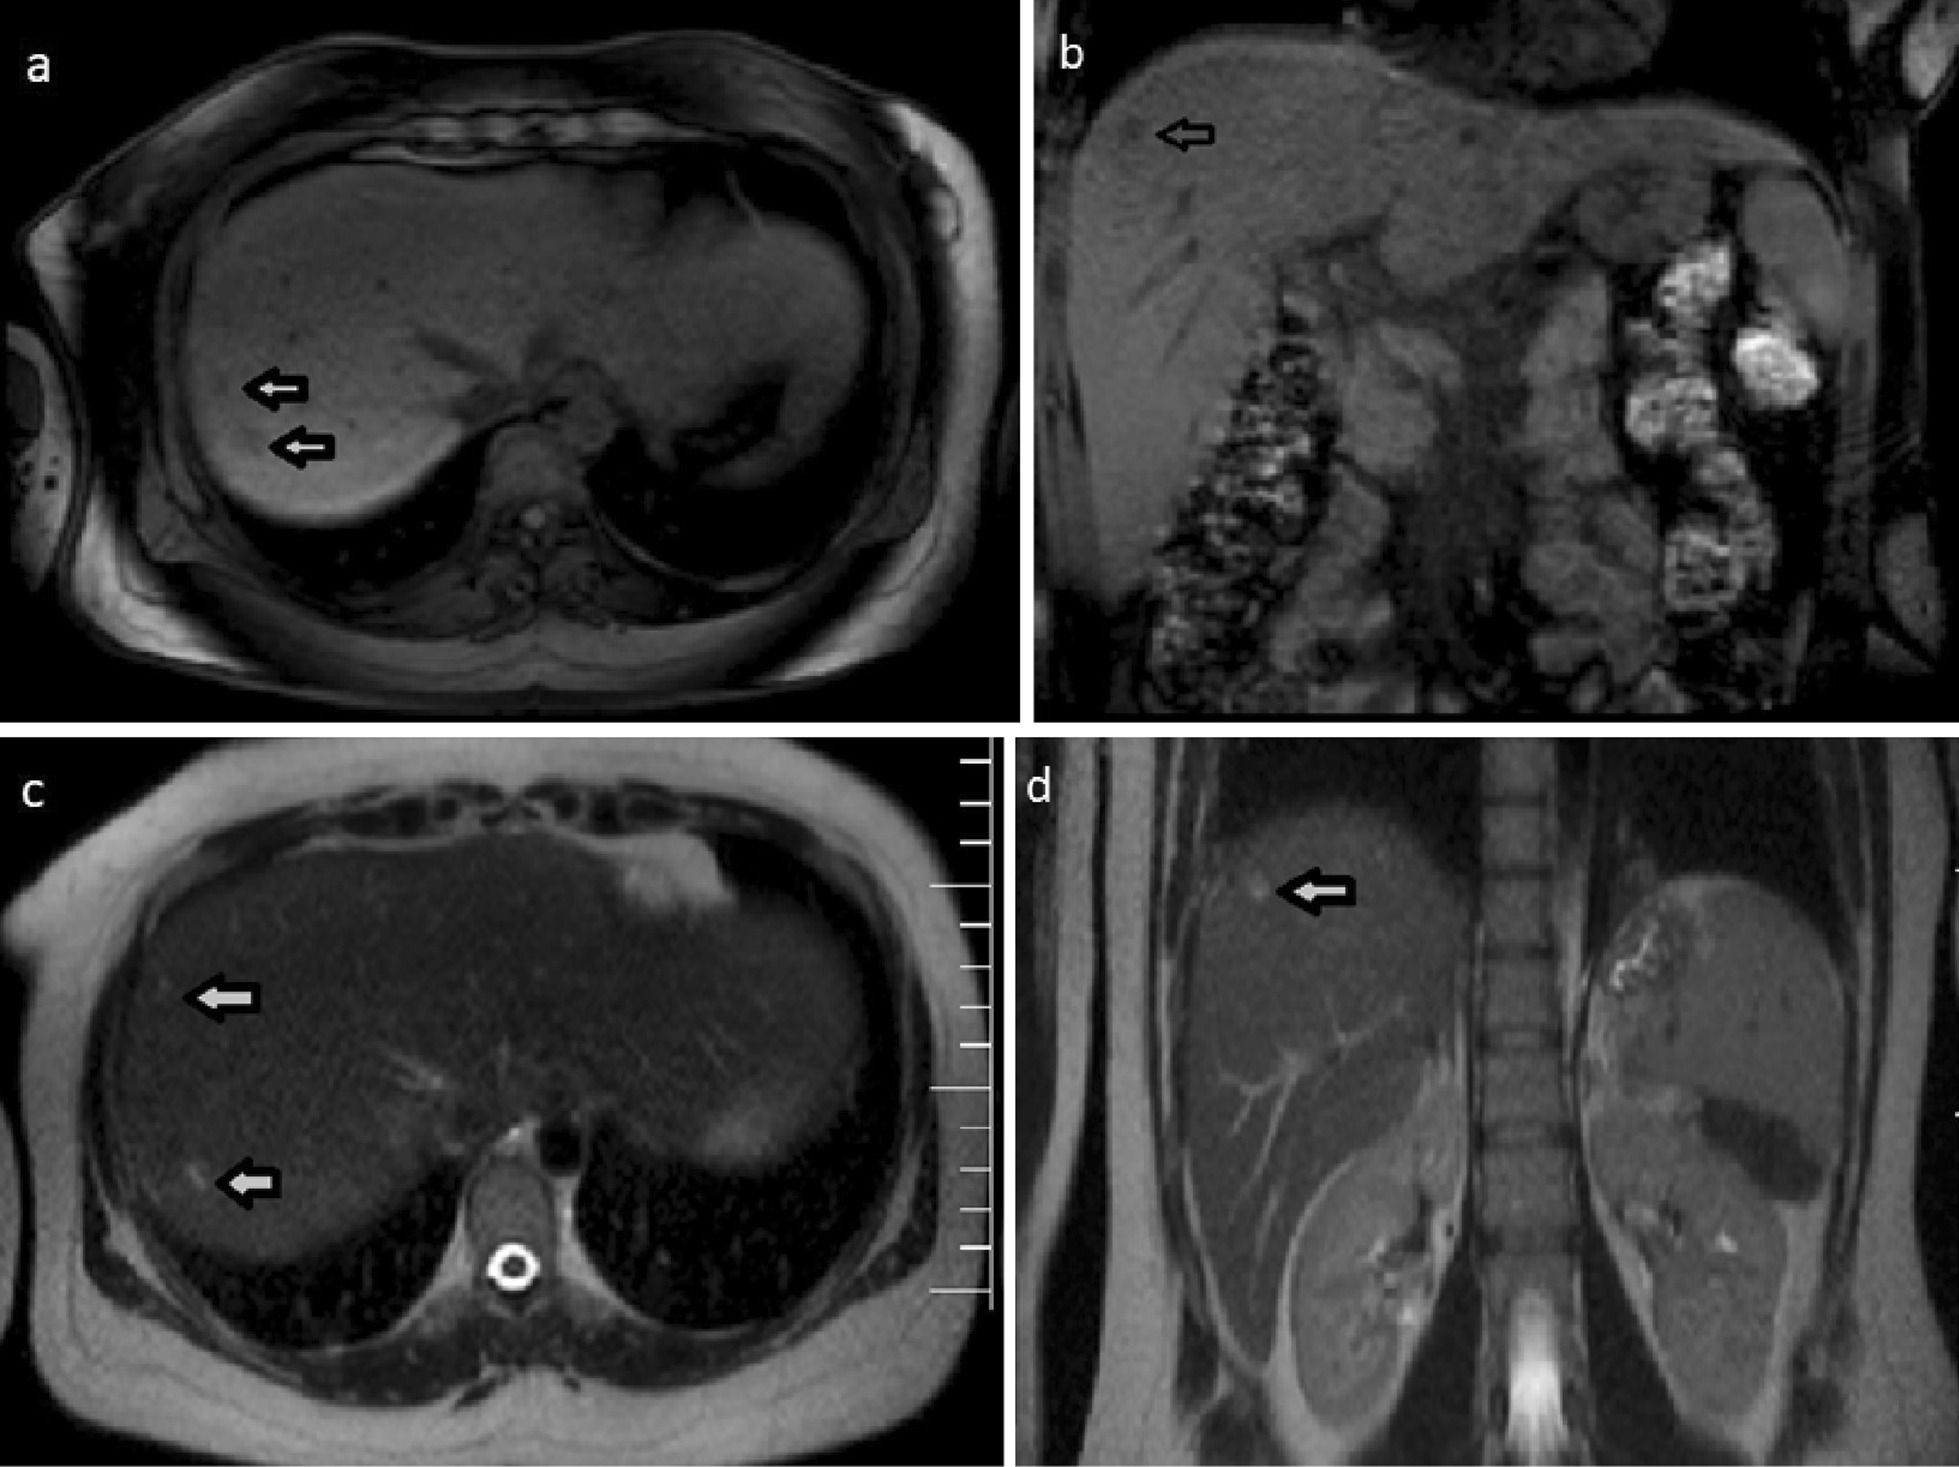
a Axial T1W MR image showing hypointense subcapsular lesions at the right lobe of the liver (arrows). b Coronal T1W MR image showing a hypointense lesion in the liver (arrow). c Axial T2W MR image showing hyperintense subcapsular/parenchymal lesions in the liver (arrows). d Coronal T2W MR image showing a hyperintense subcapsular lesion in the liver (arrow)
